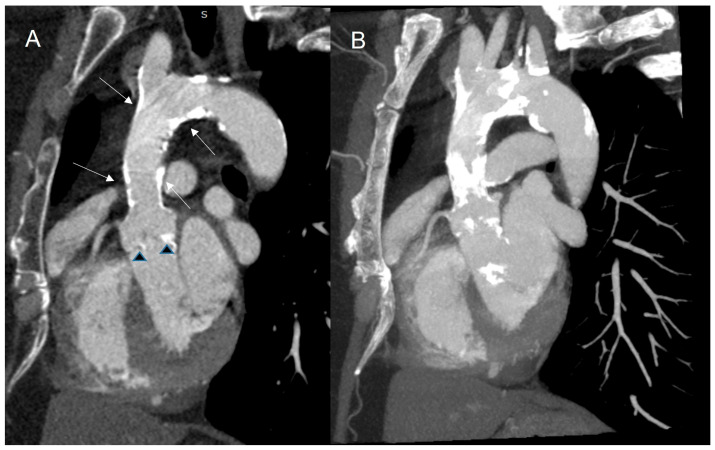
Calcifications of the ascending aorta and aortic valve after radiotherapy in a 46-year-old woman with Hodgkin’s lymphoma. (A) Oblique sagittal plane of the ascending aorta, transverse arch and proximal descending aorta. (B) Maximum intensity projection of the same plane. Extensive confluent calcifications of the ascending aorta and aortic arch—porcelain aorta (white arrows) as well as aortic valve calcification (arrowheads). CT, computer tomography.
